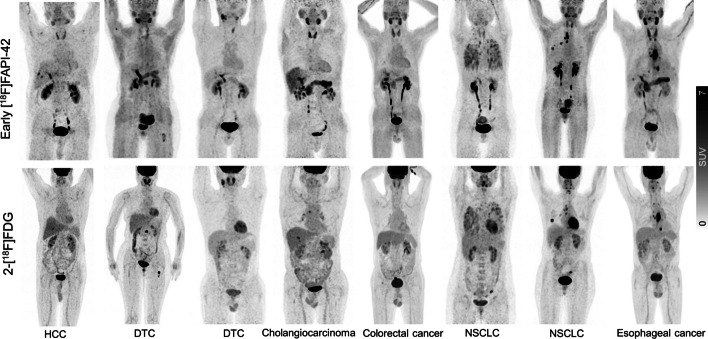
Maximum-intensity-projection images of early [^18^F]FAPI-42 and 2-[^18^F]FDG PET/CT in patients with different types of cancer. HCC = Hepatocellular carcinoma; DTC = Differentiated thyroid carcinoma; NSCLC = Non-small cell lung cancer
